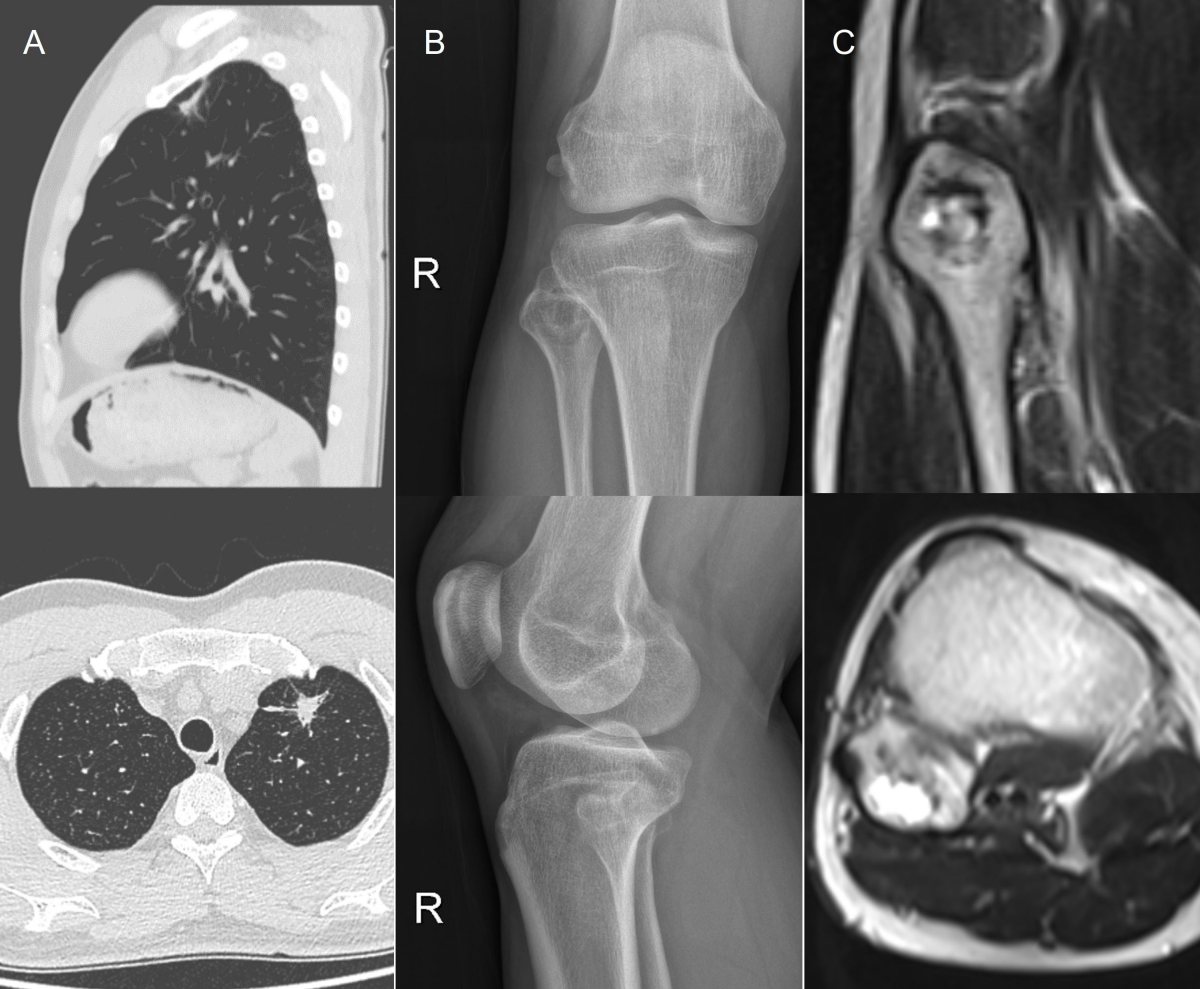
(A) Contrast-enhanced CT after Osimertinib therapy revealed a reduction in the size of the lung nodule, measuring (18mm×13mm×9mm). CT, computed tomography. (B, C) Post-radiotherapy follow-up X-ray and MRI indicated a decrease in the size of the fibular metastasis lesion, measuring (15mm×17mm). MRI, magnetic resonance imaging.
